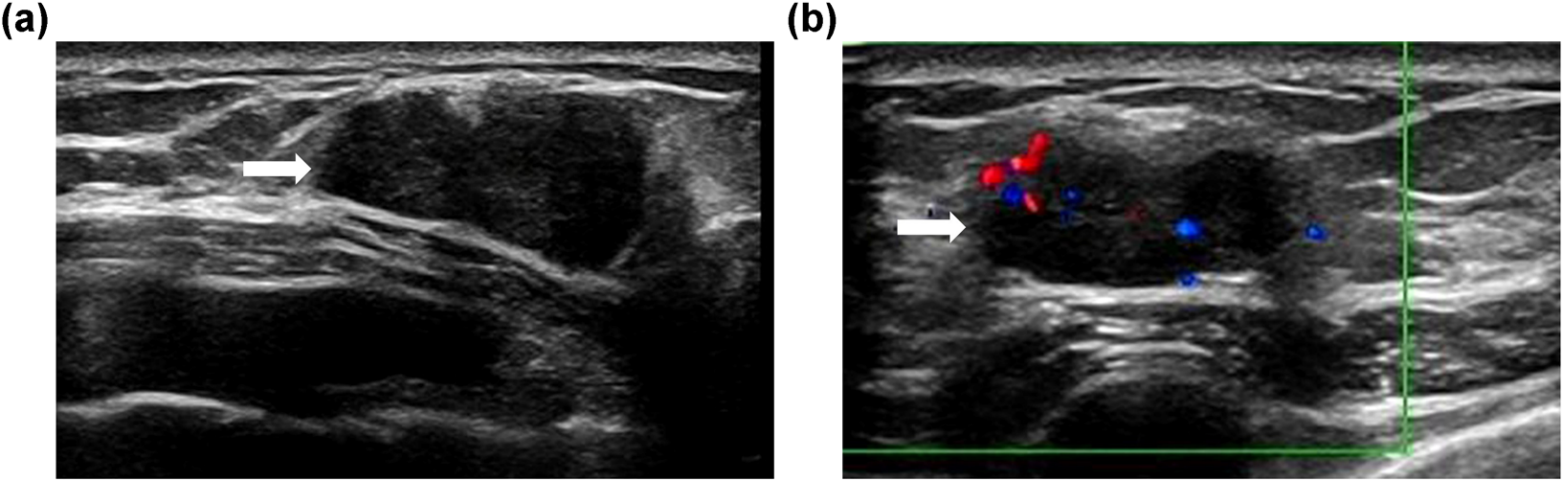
Ultrasound exam of her left breast revealed oval, parallel, circumscribed, hypoechoic masses (arrows) with internal vascularity (a and b).
